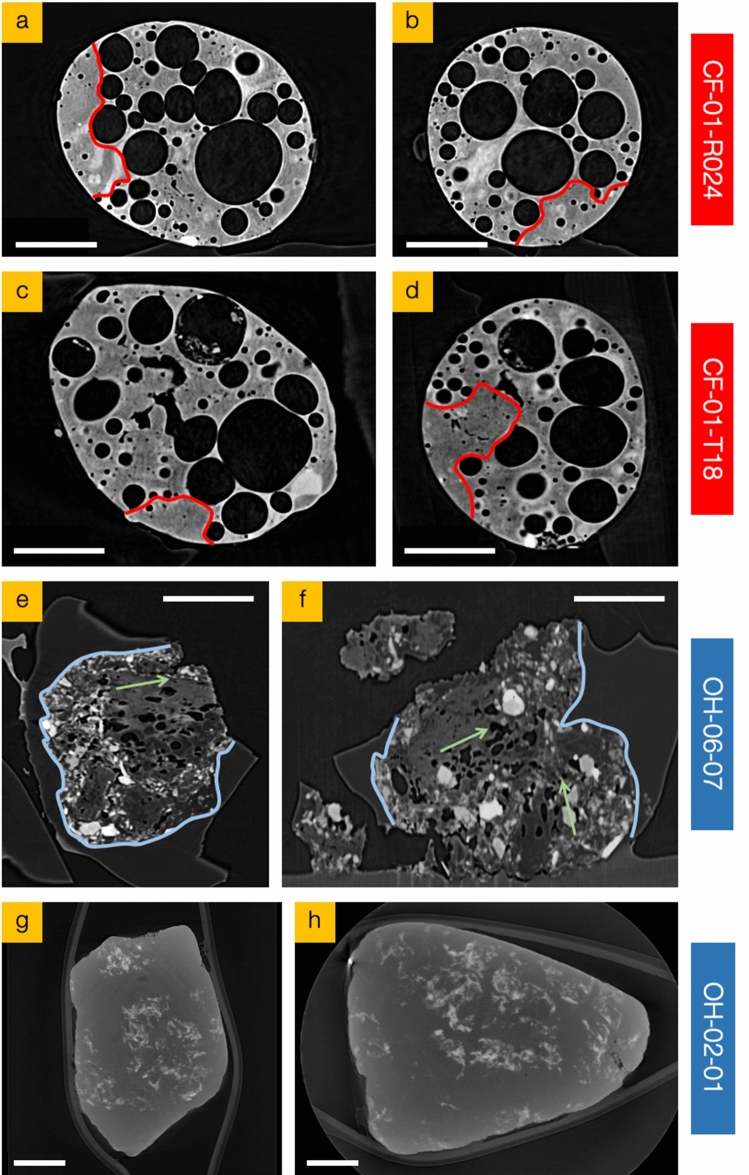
Laboratory X-ray tomography sections obtained at perpendicular orientations through the both central horizontal and vertical planes of two of the representative particulates from each suite; (a,b) CF-01-R024 (Unit 1), (c,d) CF-01-T18 (Unit 1) and (e,h) OH-06-07 (Unit 3), (g,h) OH-02-01 (Unit 3). Regions absent of voids within the representative Unit 1 particulates are red in (a)–(d). Scale bars = 100 µm.
